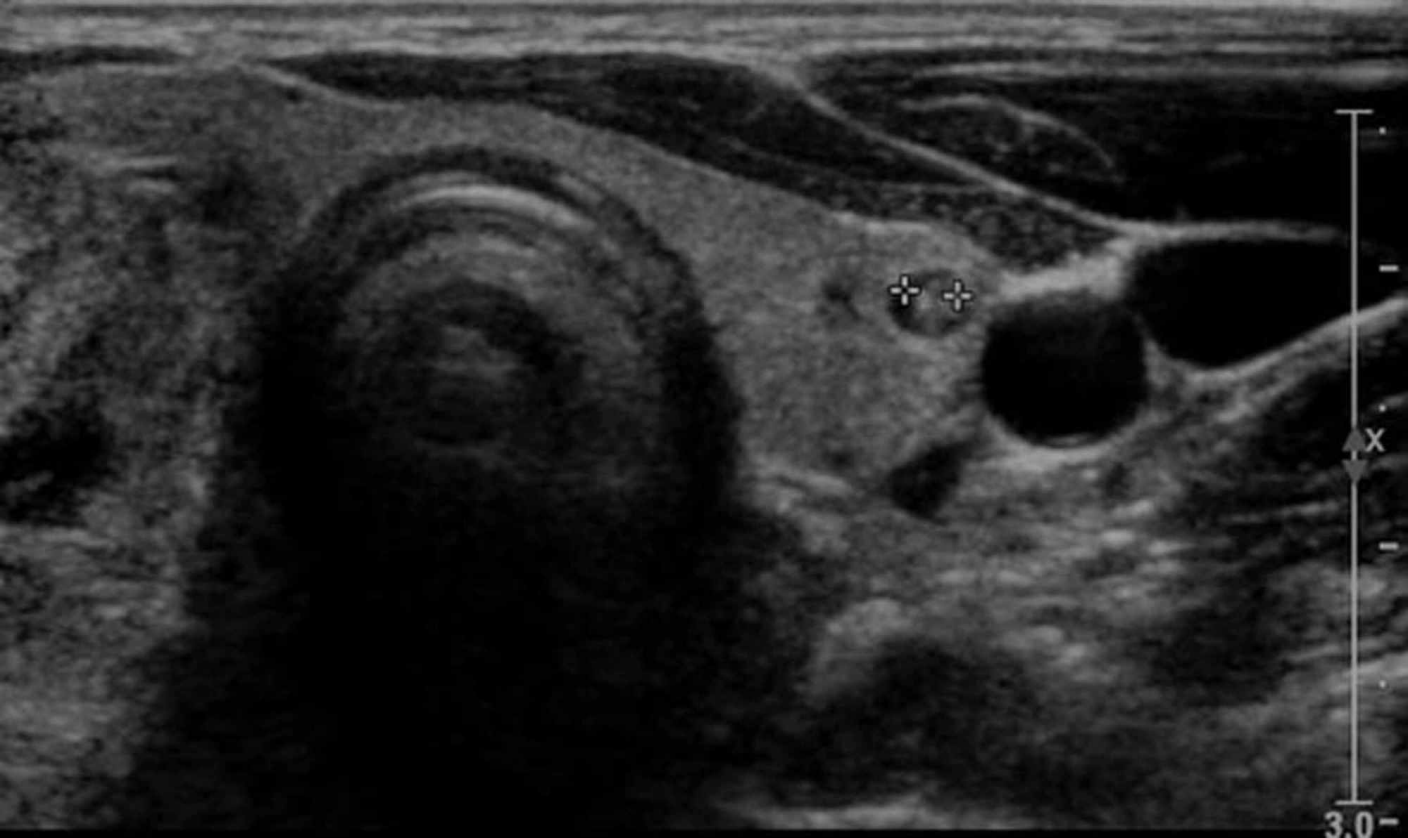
U/S of neck multinodular goiter in left side measuring 0.4 x 0.2 cm U/S, ultrasound.
